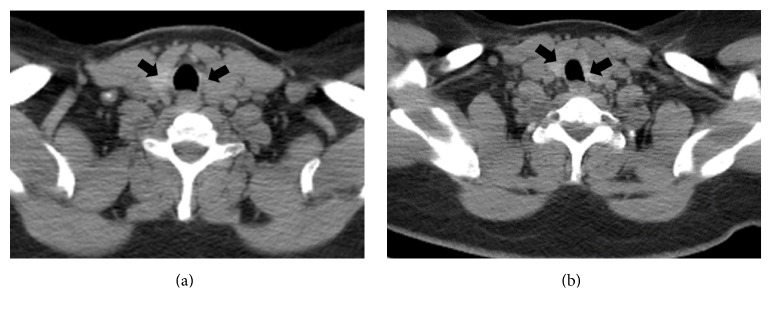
Preoperative CT scan of the neck. The preoperative CT scan of the neck shows no abnormalities. Shown are two different levels (a, b). Black arrows indicate thyroid tissue.
